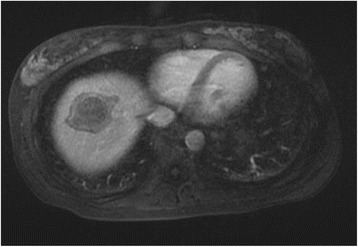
EOB-MRI at 24 weeks of gestation. Non-enhancement of a 40-mm tumor
